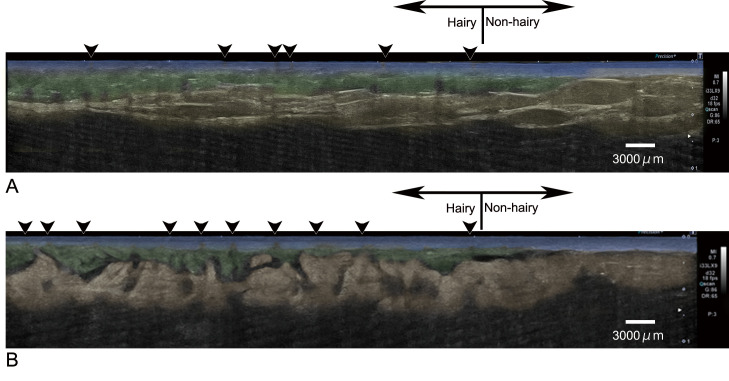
Sonographic image of skin in the axilla. Sonographic image before (A) and after (B) Lidocaine injection are shown with in color. The sweat gland layer (green) is observed as a hyperechoic area between the dermis (blue) and the fat layer (yellow) in the hairy region. Hair follicles (arrowheads) are observed as hypoechoic regions in the dermis. Subcutaneous injection of the solution created space between each tissue, making it easier to identify the sweat glands.
